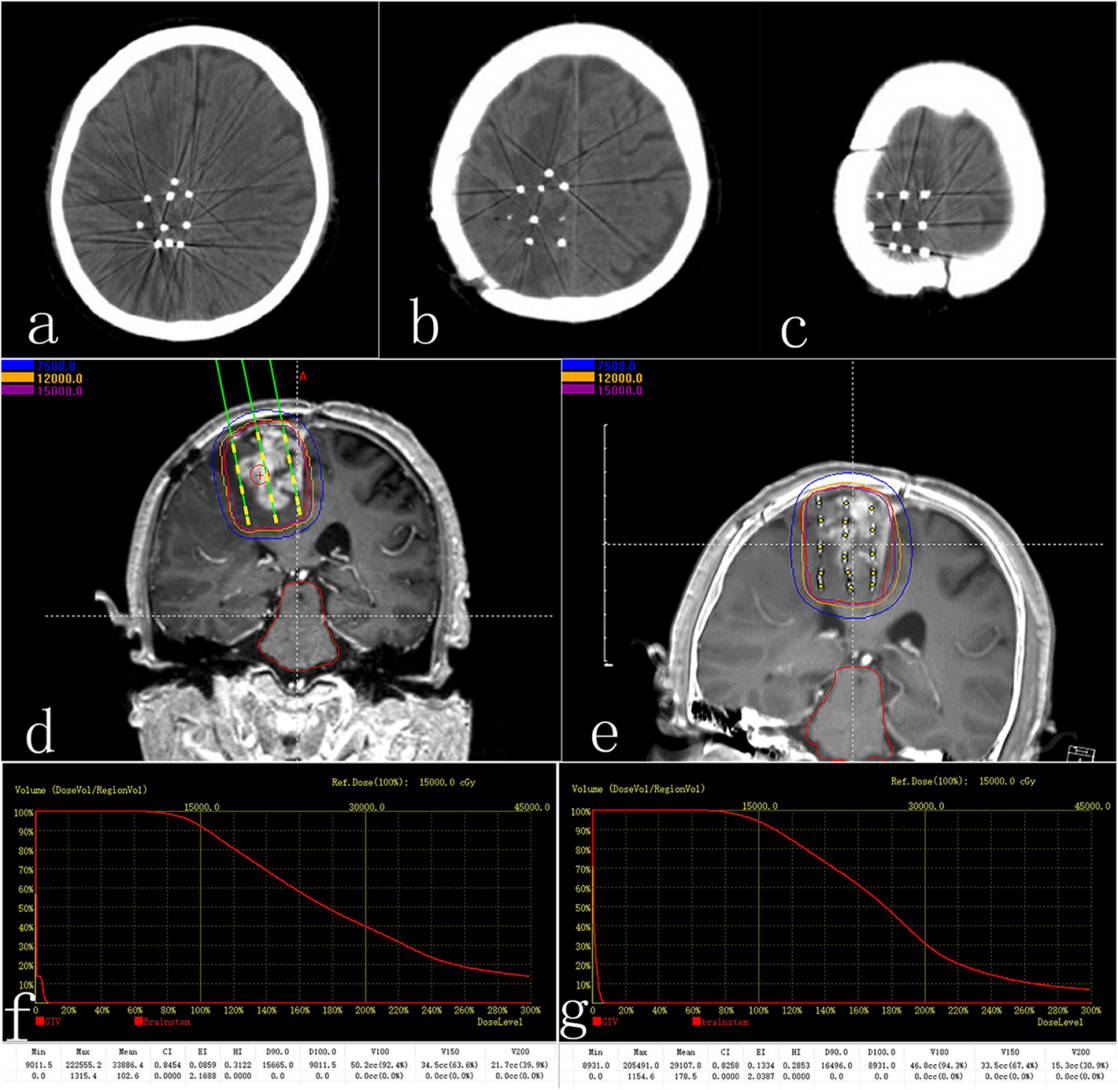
Preoperative plan and postoperative dosimetry verification. a, b, c Postoperative CT images showed ^125^I seed distribution. d Preoperative plan. e Postoperative dosimetry verification based on postoperative CT/MR fusion images. f Dose volume histogram of preoperative plan. g Postoperative dose volume histogram. Postoperative dosimetry matched the preoperative treatment plan
